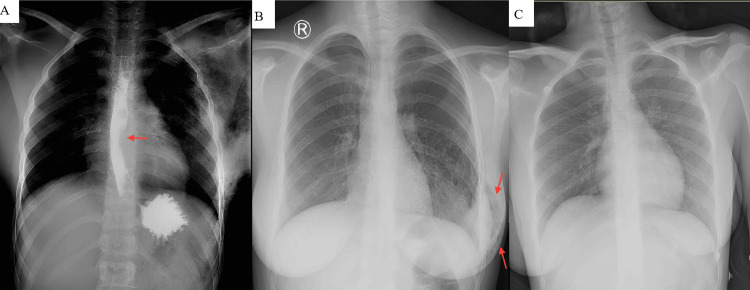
Imaging of left-sided pneumomastia with diagnostic evaluation, management, and follow-up (A) Frontal chest radiograph during fluoroscopic gastrografin swallow demonstrating free passage of contrast through the esophagus into the stomach (arrow), without evidence of contrast extravasation, fistula formation, abnormal stricture, or filling defect. (B) Posteroanterior chest X-ray following ultrasound-guided needle aspiration reveals marked reduction of left-sided pneumomastia, restoration of normal soft-tissue density, and improvement of subcutaneous emphysema, with a trace remaining (arrows). (C) Twelve-month follow-up chest X-ray confirms complete resolution of the left pneumomastia, with no evidence of recurrence in the breast or subcutaneous tissue.
